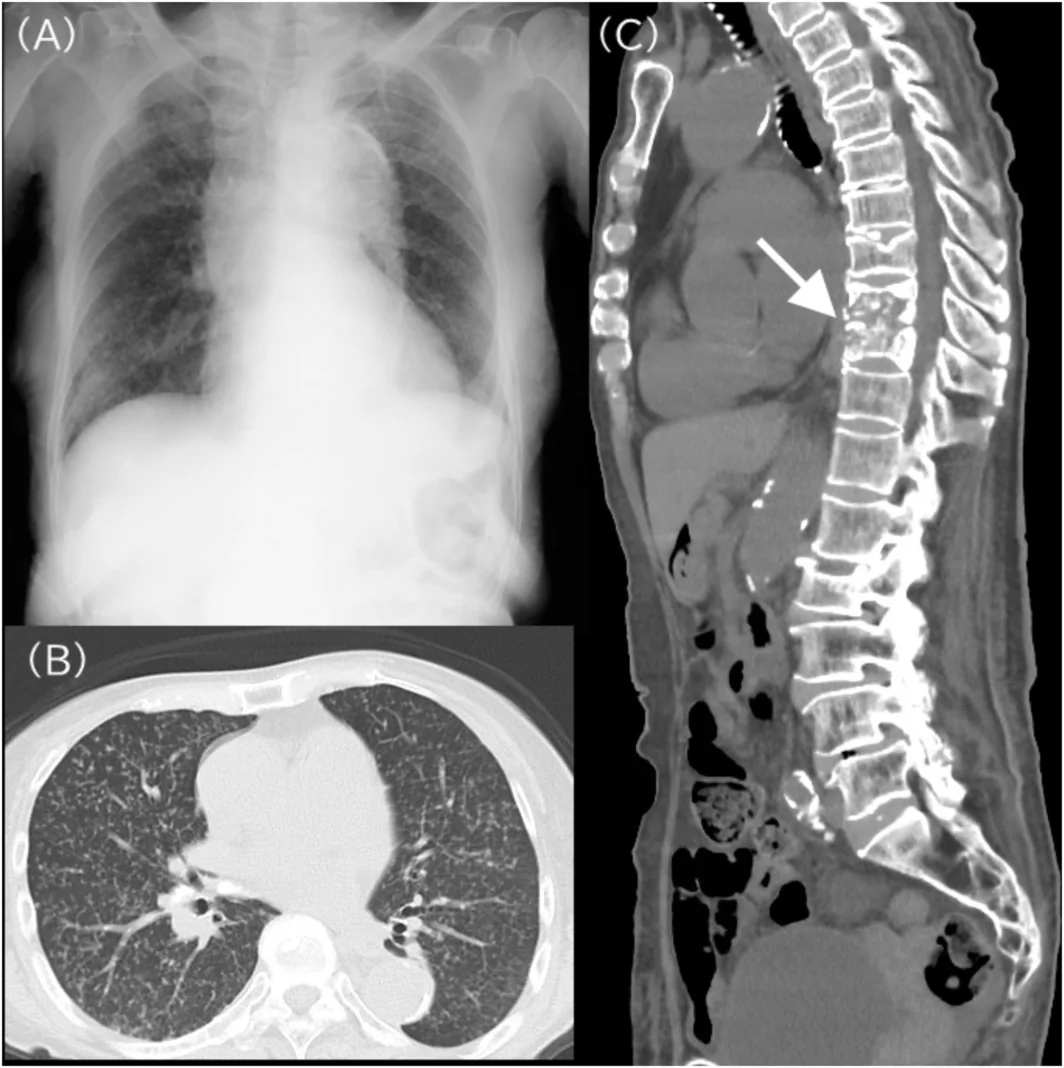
Chest radiography and computed tomography findings on admission. (A) Chest radiograph showing diffuse bilateral fine nodular opacities. (B) Chest computed tomography demonstrating diffuse bilateral finely granular pulmonary lesions consistent with a miliary pattern. (C) Sagittal computed tomography image showing destructive changes at the T9/T10 vertebral level (arrow).
